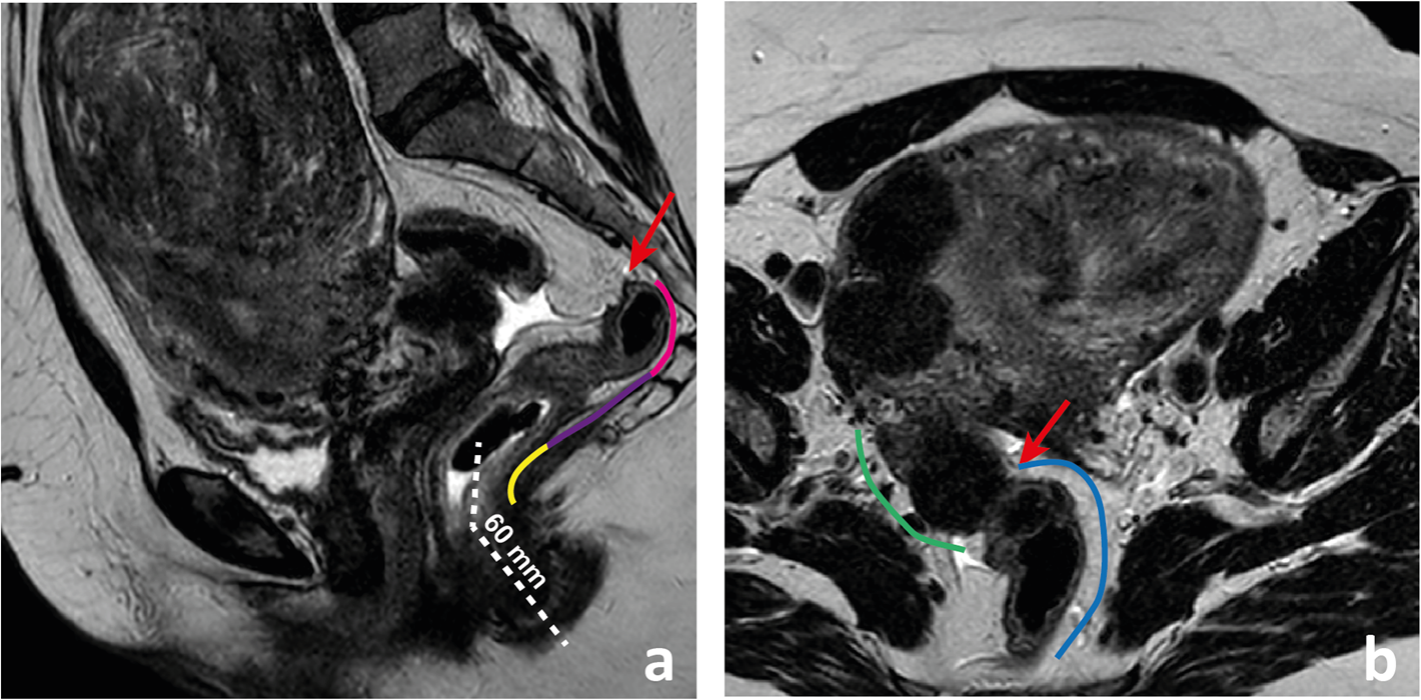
The upper limit of the rectum corresponds on MR imaging to the point of inflexion between the more vertical rectum and the more horizontal sigmoid “take off” (arrow in a). The sigmoid starts at another more horizontal inflection, better visualised in the (oblique) axial plane (arrow in b). The low rectum is the segment located < 6 cm above the anal verge and above the anorectal transition (lined in yellow in a); the mid-rectum is located between the low rectum and the plane of the lowest point of the anterior peritoneal reflection (lined in purple in a); the high rectum is the segment above it and below the sigmoid “take-off” (lined in pink in a). The sigmoid “take-off” or rectosigmoid transition is lined in blue in b, while the actual sigmoid is lined in green
